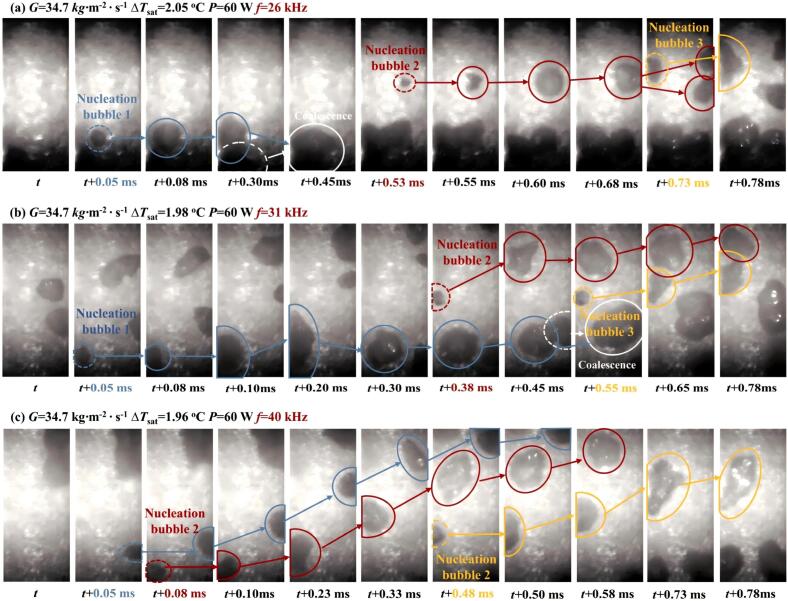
Bubble nucleation at the bottom wall with different ultrasonic frequencies [69].
